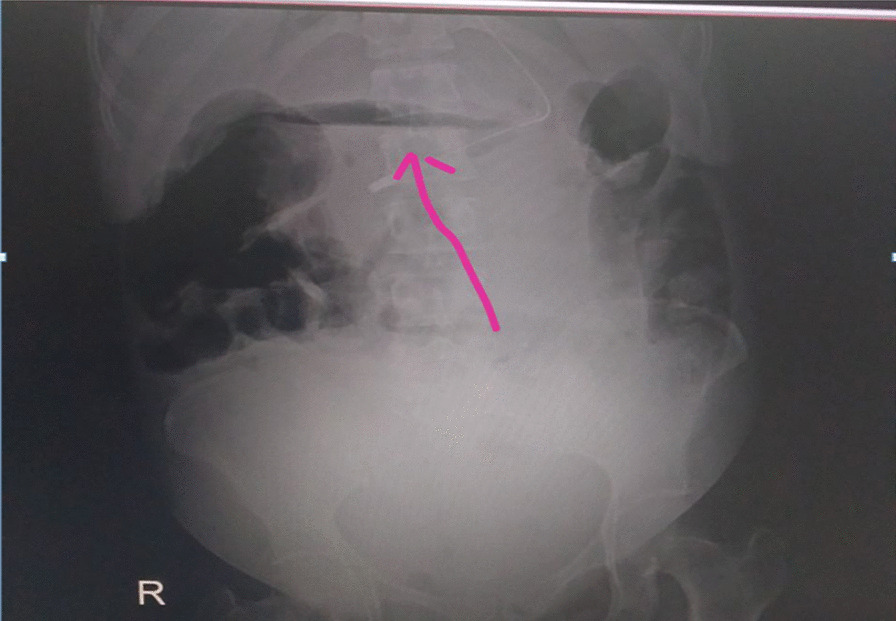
Upper abdominal X-ray taken before laparotomy showed air under the diaphragm (arrow)
